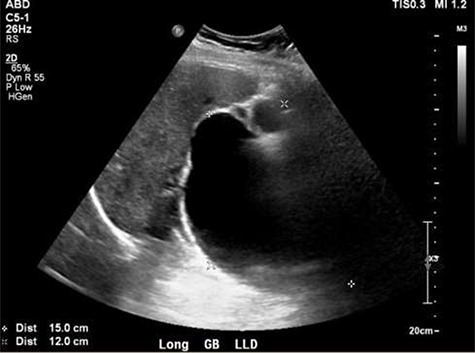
Ultrasound showing obscured CBD, 3 mm gallbladder wall, and a cystic lesion communicating with the CBD and a large gallbladder cyst.
